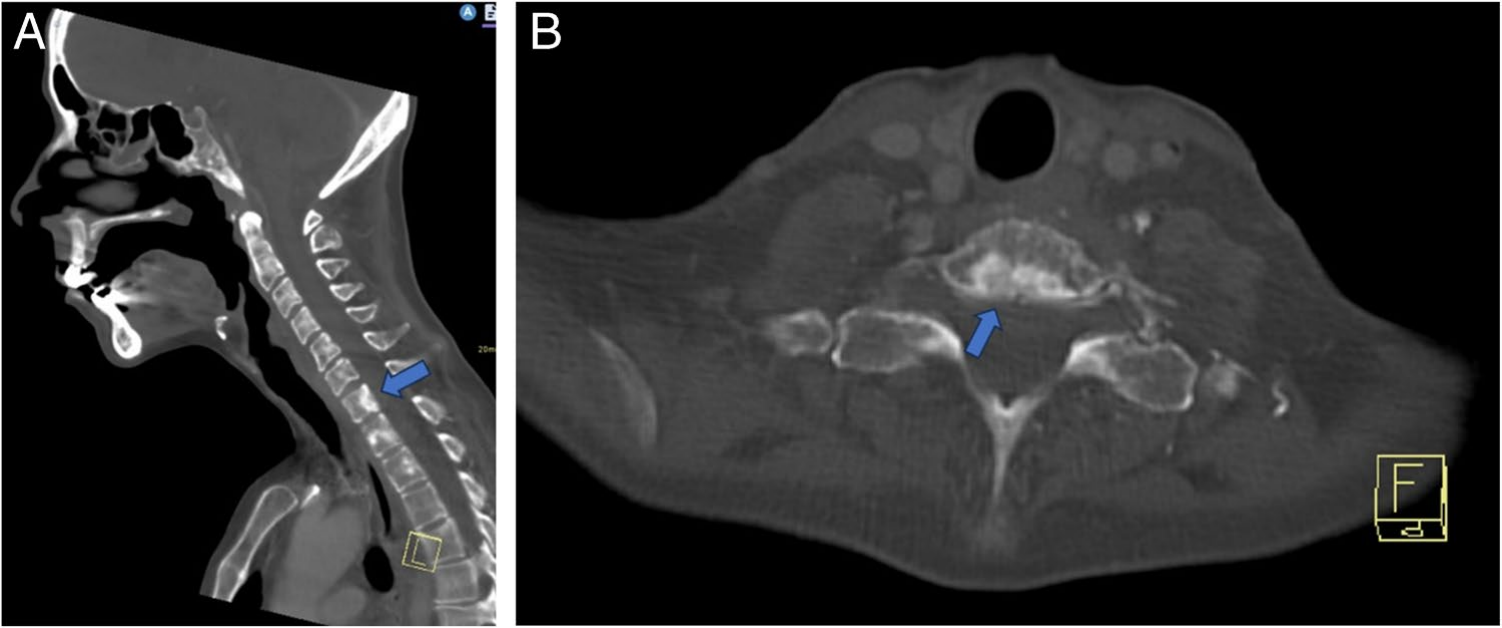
Bone algorithm sagittal midline (A) and axial (B; at the T1 level) images from contrast-enhanced neck CT demonstrate nodular hyperdensities in multiple vertebral bodies, most conspicuous in the T1 vertebral body (arrows)
